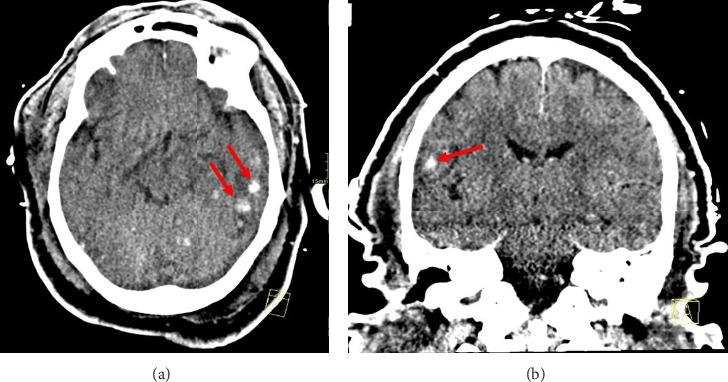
CT scan of the head. (a) Computed tomography (CT) head without contrast showing multiple peripherally located subcentimeter hemorrhages. (b) Computed tomography (CT) head with IV showing parenchymal hemorrhages.
